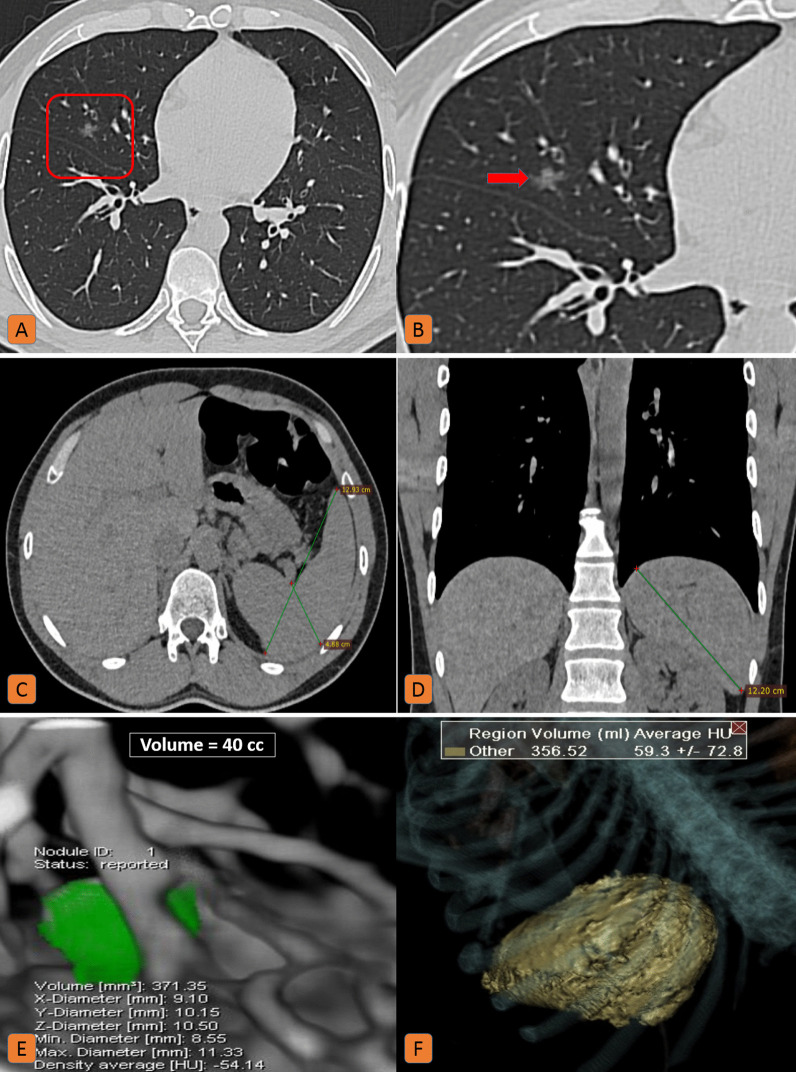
Splenomegaly in a 35-year-old male patient with mild COVID-19 infection. A Axial chest CT (lung window) and B zoomed image showed single right middle small ground-glass nodular opacity. C Axial, and D coronal CT cuts of the upper window (soft tissue window) showed splenic 2D diameters (13 × 4.6 × 12.4 cm) (anteroposterior diameter x side to side thickness x craniocaudal diameter). E Lung volumetric CT assessment revealed a 40 cc lung nodule. F CT volumetry of the spleen was 356 cc
